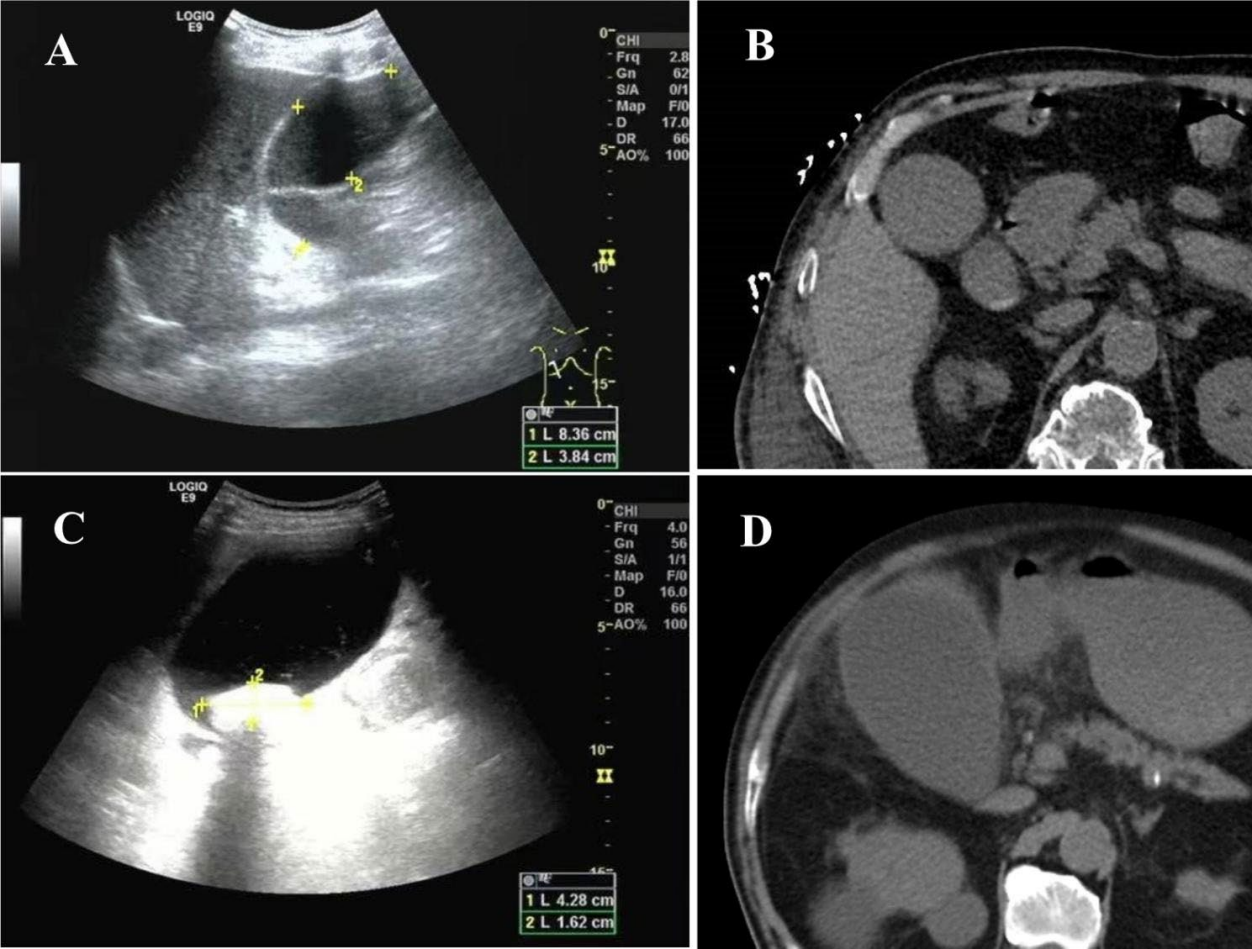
Abdominal ultrasound and CT Findings in two cases A. The ultrasound results of case 7 revealed that there was gallbladder enlargement with no stones. B. The abdominal CT of case 7 revealed the presence of gallstones. C. In case 9, abdominal ultrasound revealed gallstones with gallbladder enlargement. D. In case 9, no stones were found in the abdominal CT.
